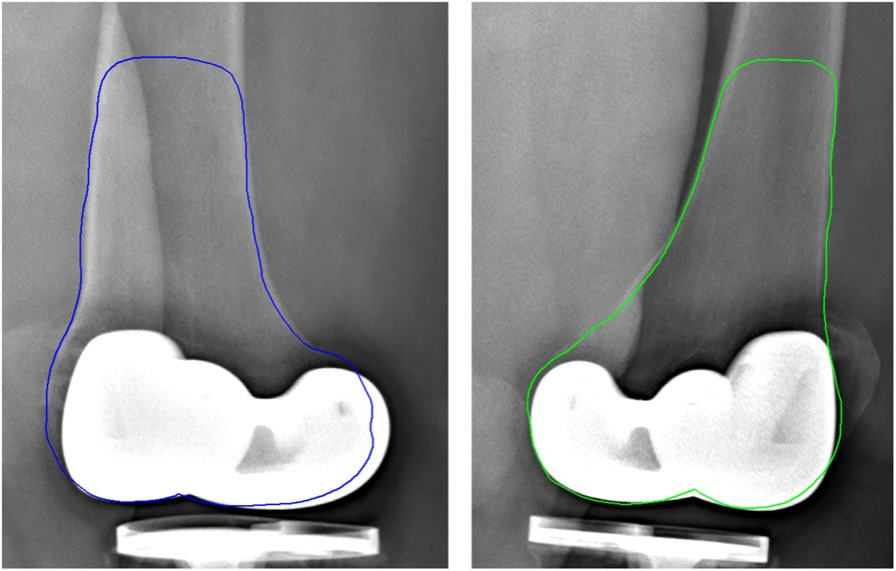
Example of our registration for the distal femur on real radiographic images : 45-degree image (left) and 135-degree image (right)
